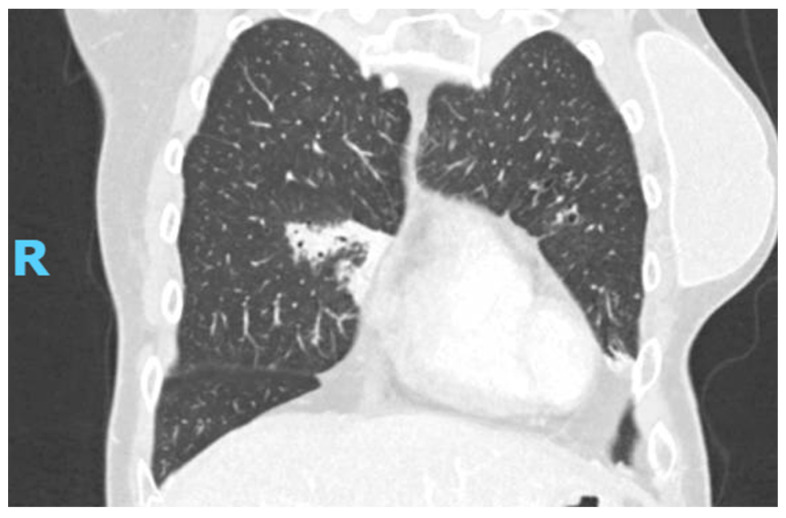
Follow-up chest CT scan revealed persistent inflammatory opacities without new consolidations.
